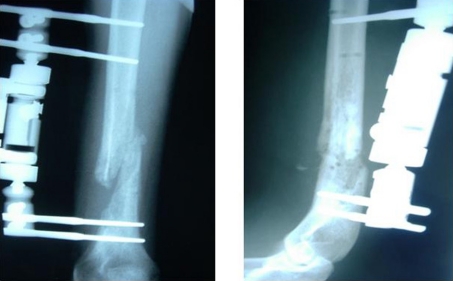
AP and lateral x-rays demonstrating the stabilization of the fracture of the distal humerus by means of a monolateral external fixator.
